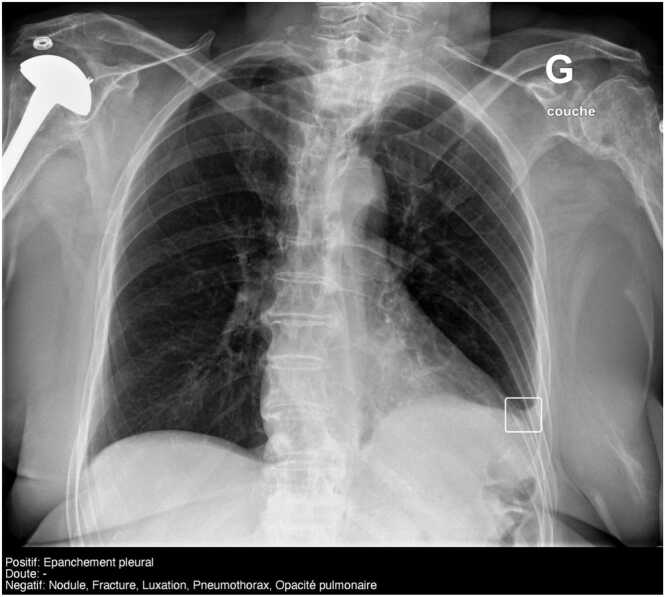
Example of a chest discordant case correctly diagnosed by AI. Frontal view of chest radiograph in adult, as part of a multiple bodyparts examinations (including hip and knee, reported as normal). The chest was reported as normal by the ED. The radiologist reported a minimal left pleural effusion. AI output shows positive pleural effusion (white solid bounding box). French translation: doute = doubt; opacité pulmonaire = lung parenchymal opacity; luxation = dislocation; negatif = negative; positif = positive.
